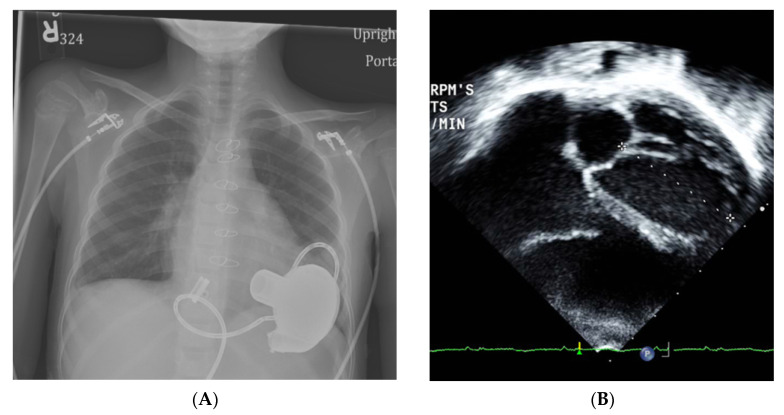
Myocardial recovery after VAD implant. (A) Chest-X-ray ~eight months post VAD implant showing resolution of cardiomegaly. (B) Post VAD implant, trans-thoracic echocardiogram in the apical four-chamber view showing normal LV dimensions with mildly depressed systolic function, LVEF 51%.
